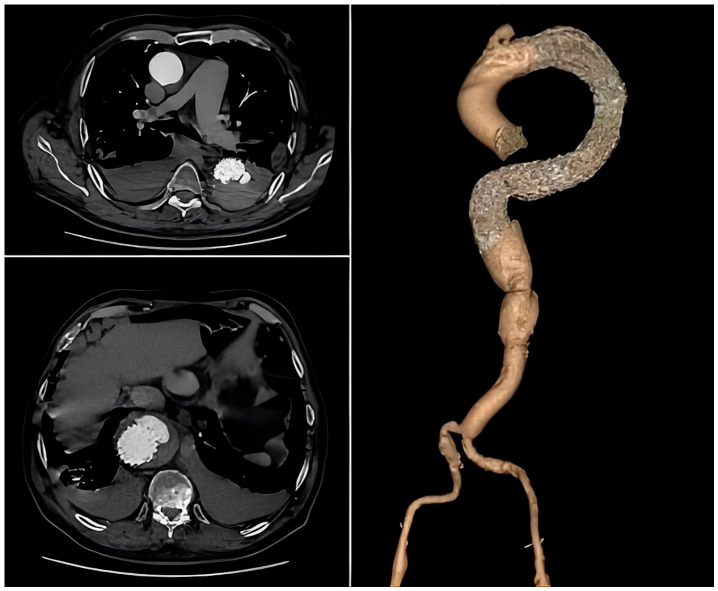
Follow-up CT angiography demonstrating post-procedural endoleak after endovascular aneurysm repair. Persistent contrast opacification outside the endograft is consistent with an endoleak, with residual aneurysmal sac perfusion. These findings highlight the role of CTA in post-treatment surveillance and detection of complications.
